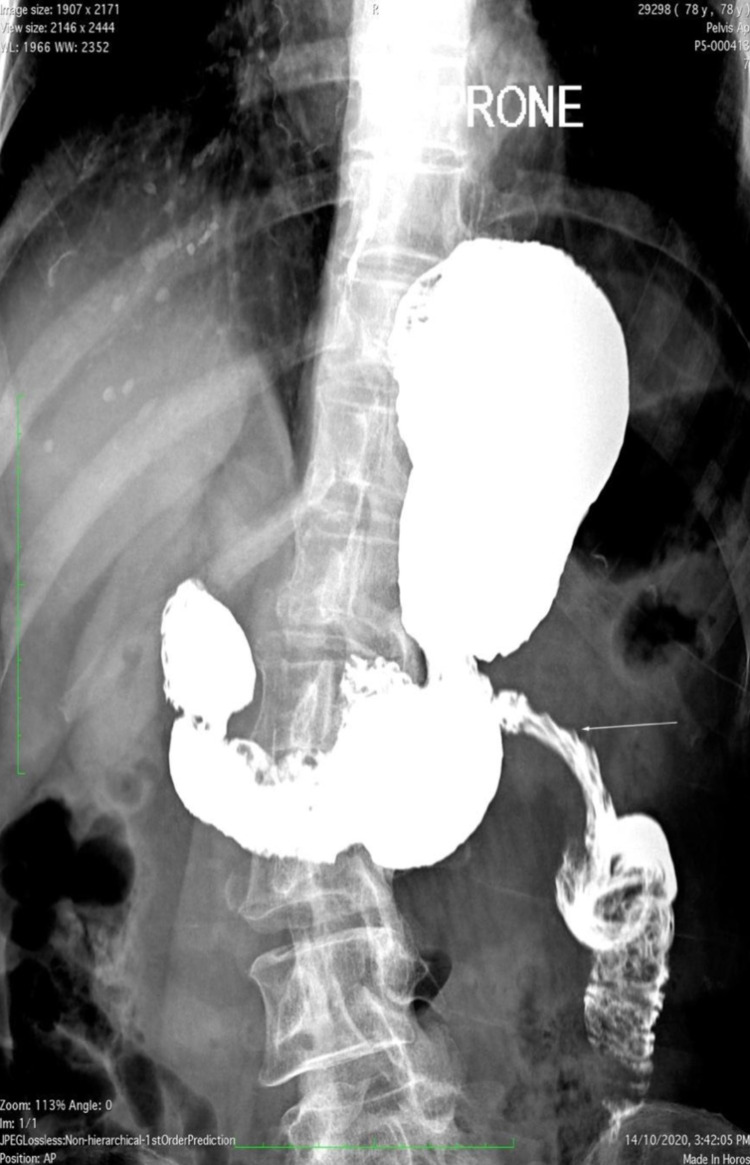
Prone view of a barium follow-through study demonstrating a focally narrowed segment of proximal jejunum at the area of the CT detected jejunal intussusception, consistent with reactive mural edema There is no tight stricture, with no evidence of a mass or filling defect.
